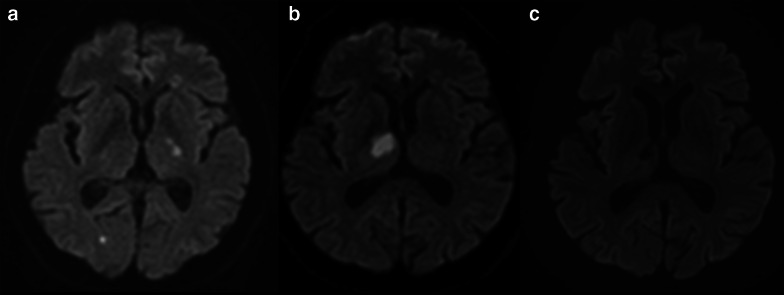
Brain diffusion-weighted magnetic resonance images. a multiple cerebral infarctions (right occipital lobe, left internal capsule putamen). b Cerebral infarction recurrence (internal capsule of the right thalamus) seen 1 month later. c No cerebral infarction 6 months postoperatively
